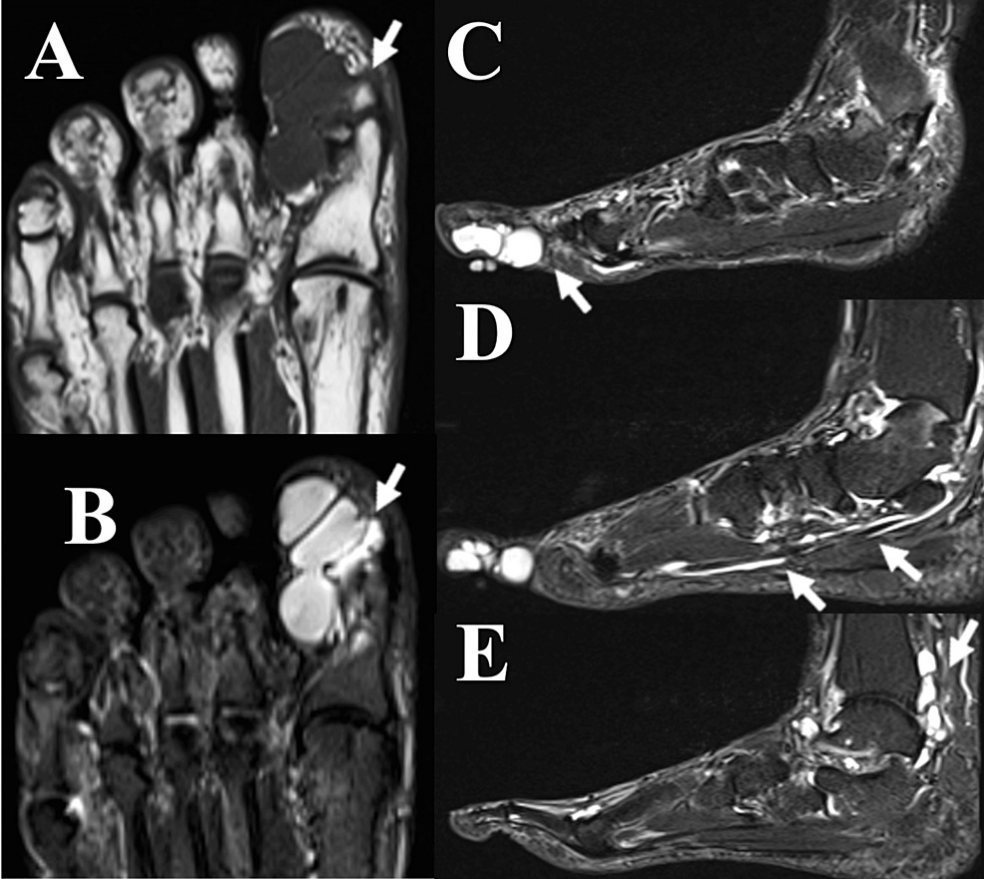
Findings on MRI (Case 1) (A-B) Axial T1-weighted (upper) and short tau inversion recovery (lower) sequences show a multi-cystic nodular lesion on the plantar aspect of the hallux. (C–E) MRI of the left ankle demonstrating short tau inversion recovery sequences in the sagittal plane. Along with the cysts, fluid accumulation was observed within the FHL tendon sheath and ankle joint. MRI: magnetic resonance imaging, FHL: flexor hallucis longus
